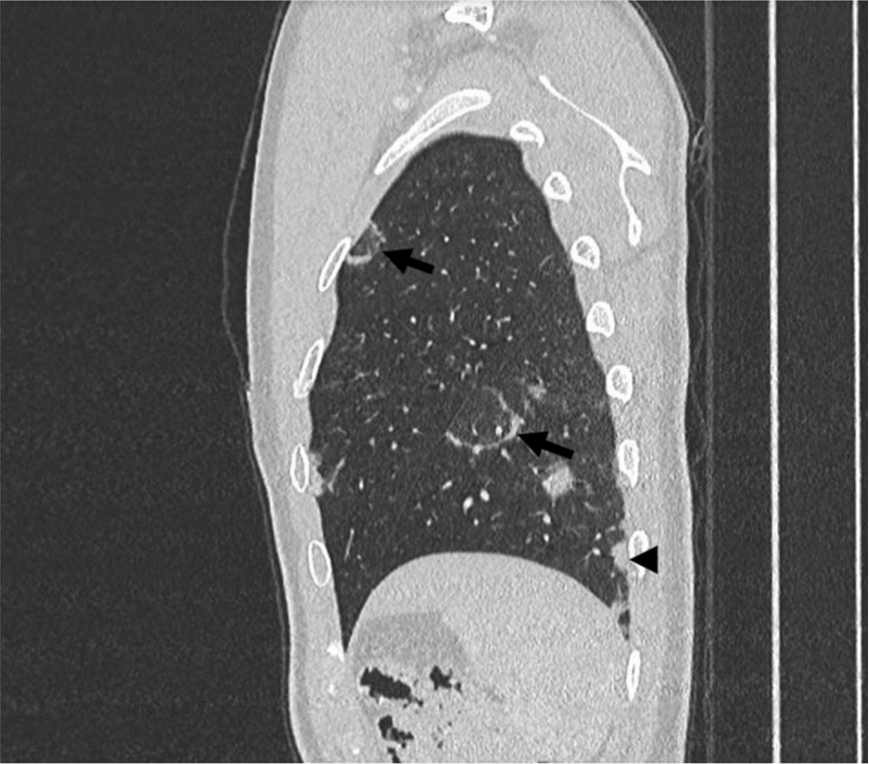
Sagittal CT chest of a 23‐year‐old patient with GPA showing multiple ill‐defined lung nodules affecting both upper and lower lobes, also with subpleural involvement (arrowhead). The Atoll sign is present as a ground‐glass nodule being surrounded by a thin rim of peripheral consolidation (arrows).
